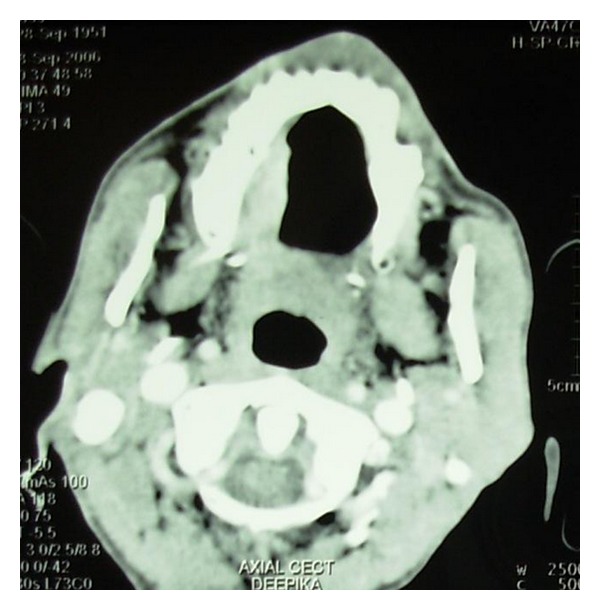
Axial CT scan showing ill-defined enhancing soft tissue mass on right side of hard palate extending posteriorly upto the soft palate.
